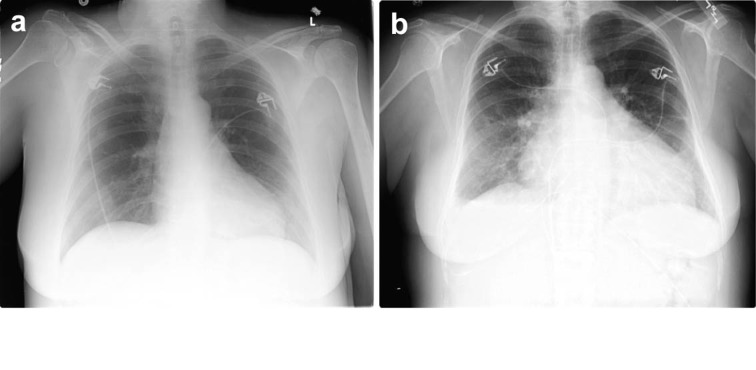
(a) Routine chest X-Ray at the diagnosis of MM; (b) Cardiomegaly 3 months after.
